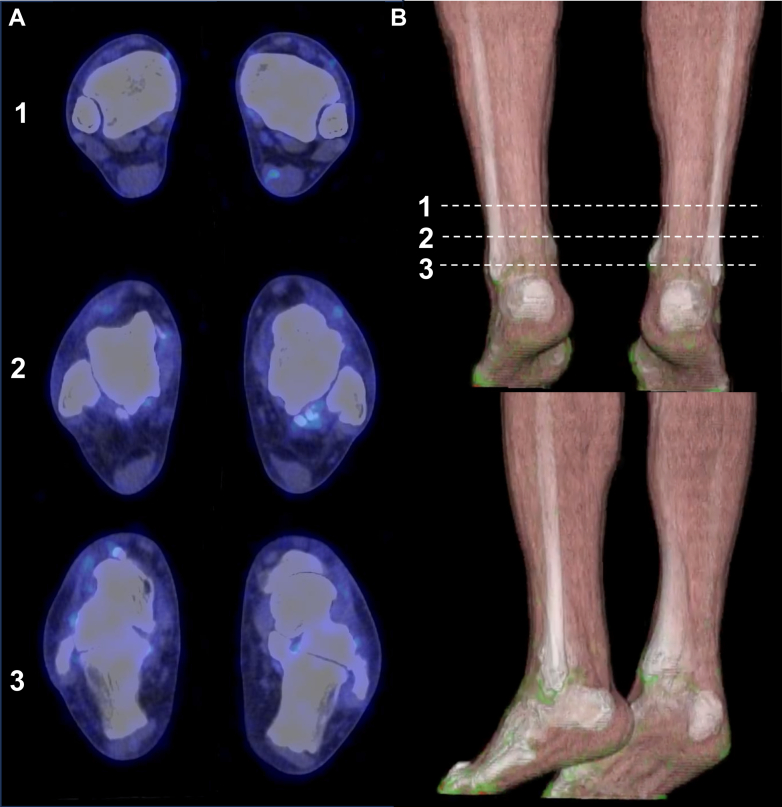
FDG-PET/CT of Achilles Tendons in the 71-Year-Old Father FDG-PET/CT showing no significant tracer uptake in either Achilles tendon, indicating absence of detectable inflammatory activity. FDG-PET/CT = ^18^F-fluorodeoxyglucose positron emission tomography/computed tomography.
